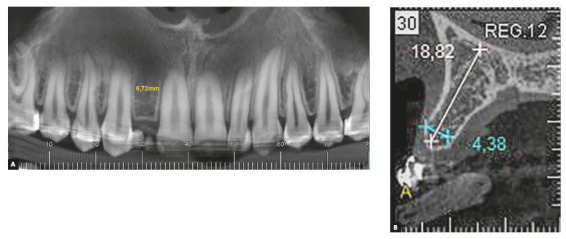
Cone-beam CT slices. Panoramic view shows interradicular distance
